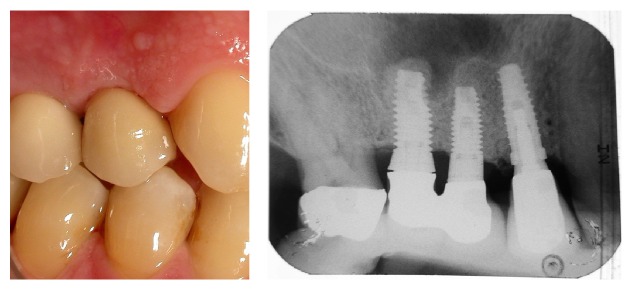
Case 1: 6 years of follow-up and radiographic control.
